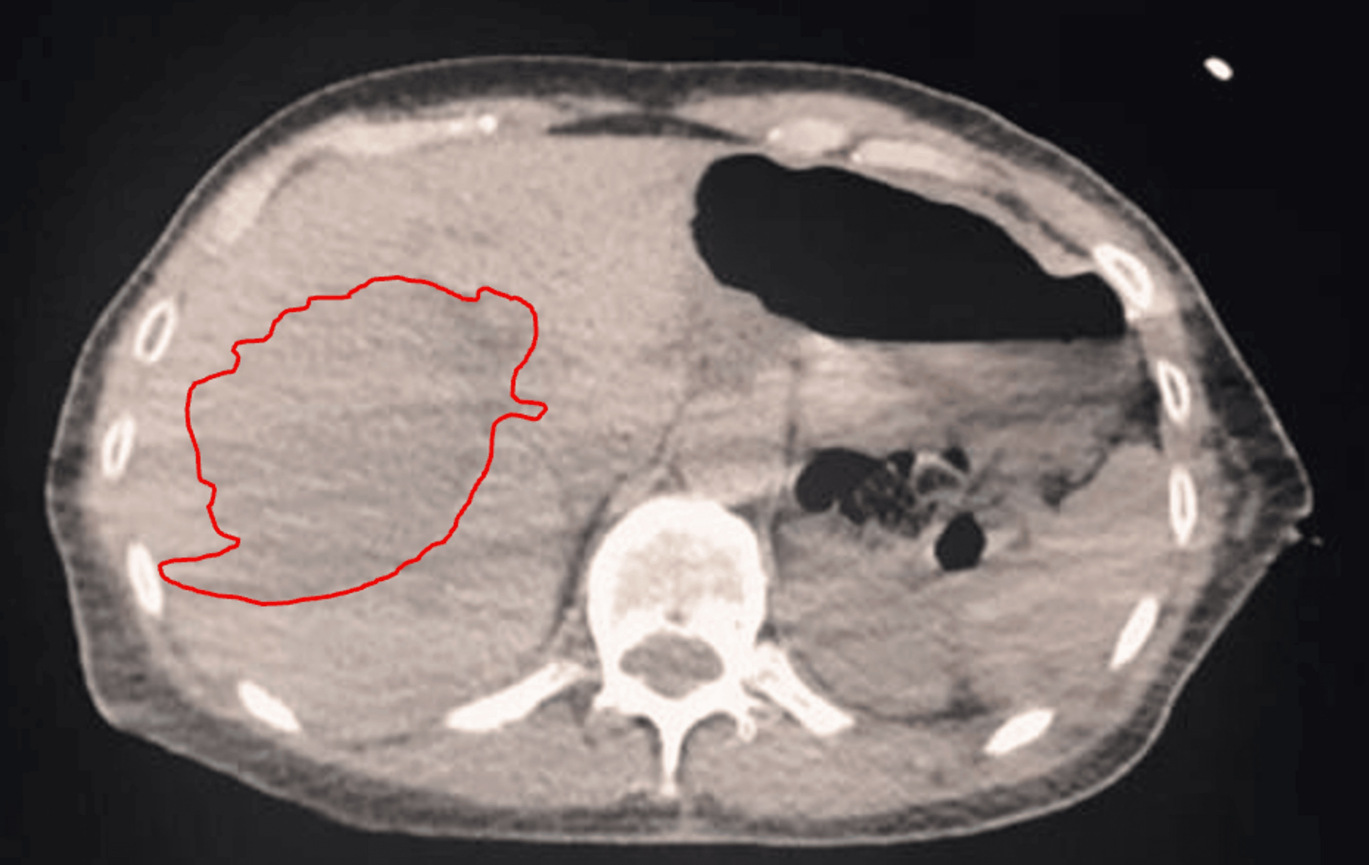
Initial axial CT of the abdomen demonstrates a hemangioma (red tracing) measuring 8.2 x 6.4 cm.
